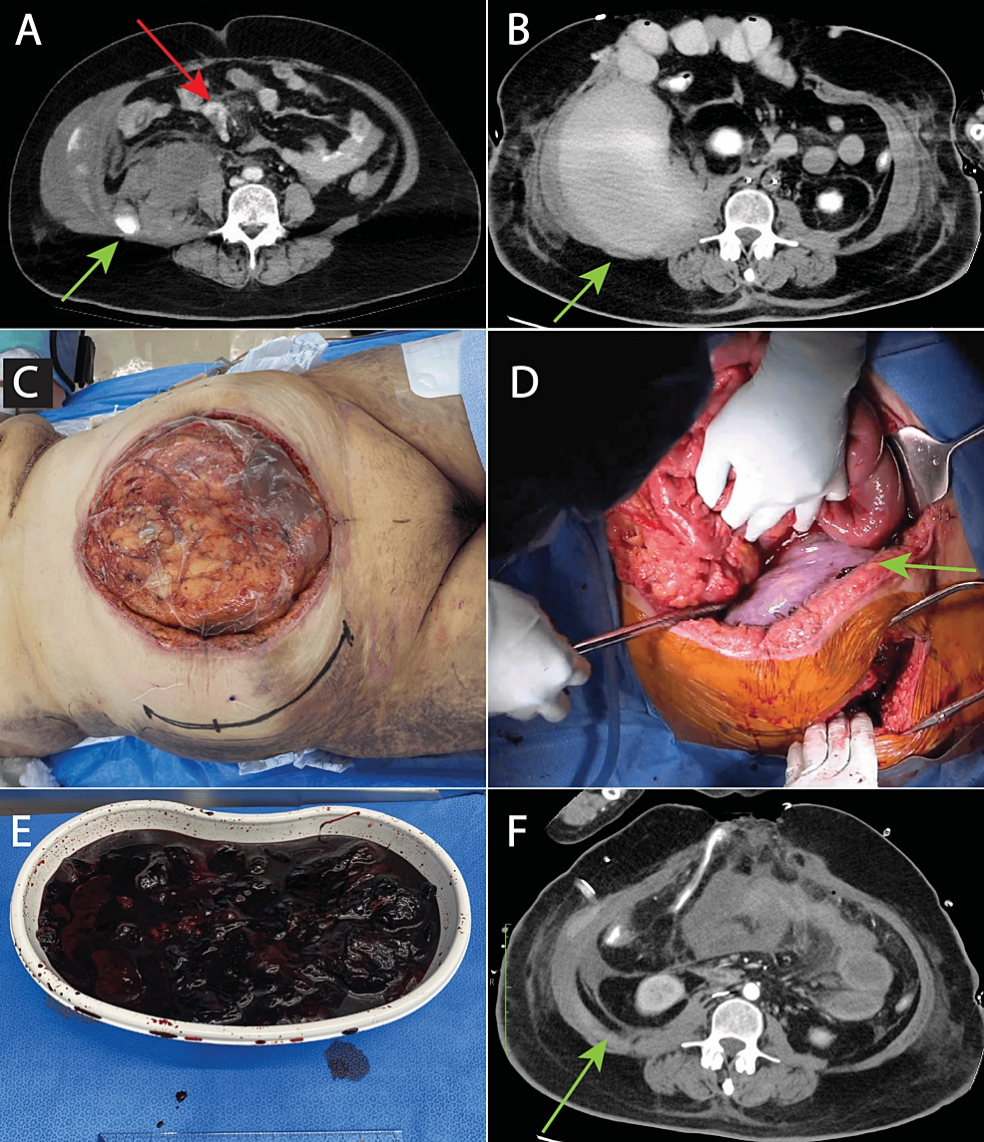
Perioperative findings (A) Preoperative computed tomography scan obtained at a nearby hospital after trauma showing hemorrhage from a damaged mesenteric artery (red arrow) and retroperitoneal hemorrhage (green arrow). (B) A post-laparotomy computed tomography scan showing a massive hematoma in the right retroperitoneum (green arrow). (C) Preoperative appearance of the patient before retroperitoneal surgery; the abdomen is open with the location of the parabdominal rectus muscle marked. The patient's cephalic side is on the left and her caudal side is on the right. (D) The left retroperitoneum was opened intraoperatively, and intraperitoneal observation revealed a bulging right retroperitoneum. (E) A dark red hematoma weighing 1200 g was manually removed. (F) Postoperative computed tomography scan showing marked reduction of the hematoma in the right retroperitoneum.
